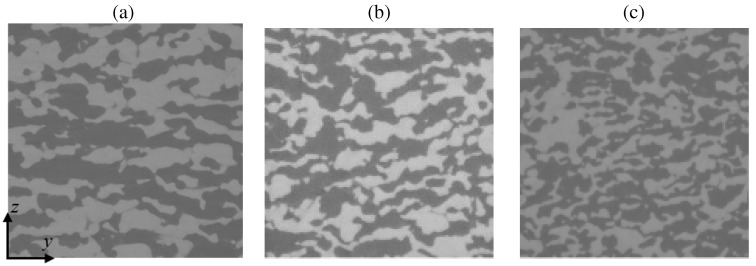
CT scan of the three ceria samples of Table 1: (a) No. 1; (b) No. 2; and (c) No. 3. Edge length of the pictures is 376 *μ*m. Dark is solid phase and bright is void phase.
